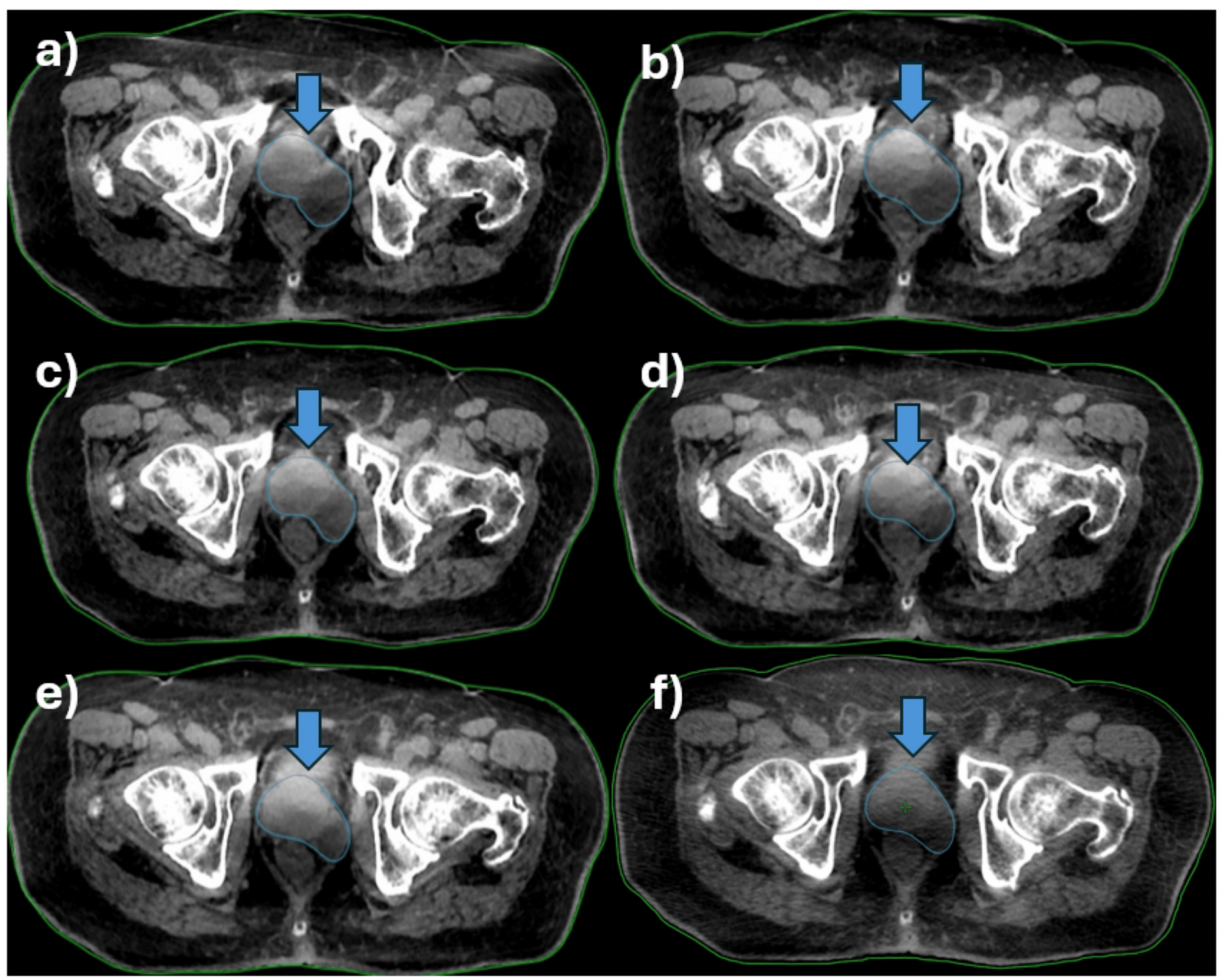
Weekly response of the primary tumor in the prostate during daily OART as seen on CBCT images acquired in a) first week, b) second week, c) third week, d) fourth week, e) fifth week, and f) last day of treatment. CTVp (blue arrow) is contoured using a blue outline. OART: online adaptive radiotherapy, CBCT: cone beam computed tomography, CTVp: clinical target volume of the primary tumor
